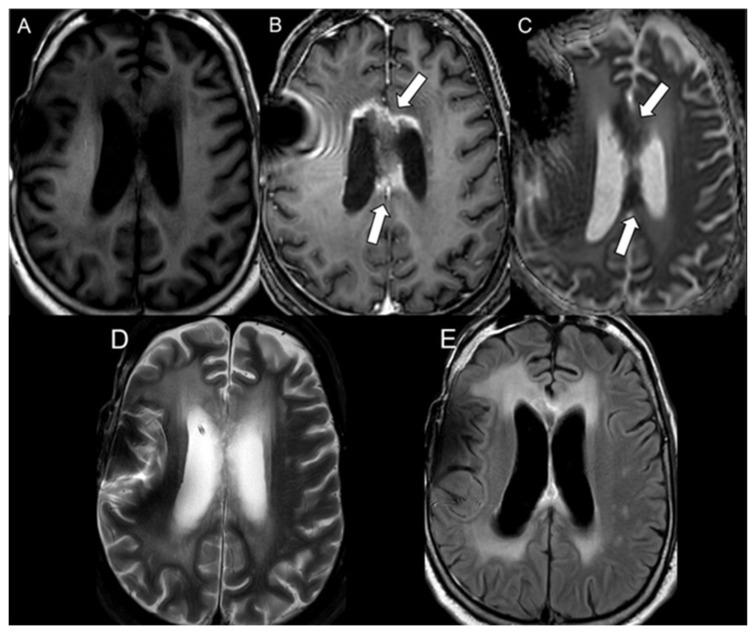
A 61 y/o male with radiation necrosis. Axial MRI of the brain including (A) T1 weighted image, (B) T1 weighted postcontrast image, (C) ADC map, (D) T2 weighted image, and (E) FLAIR. The lesion demonstrates some contrast enhancement within the body of the corpus collosum and fornix (white arrows in (B)) and restricted diffusion on the ADC map (white arrows in (C)).
